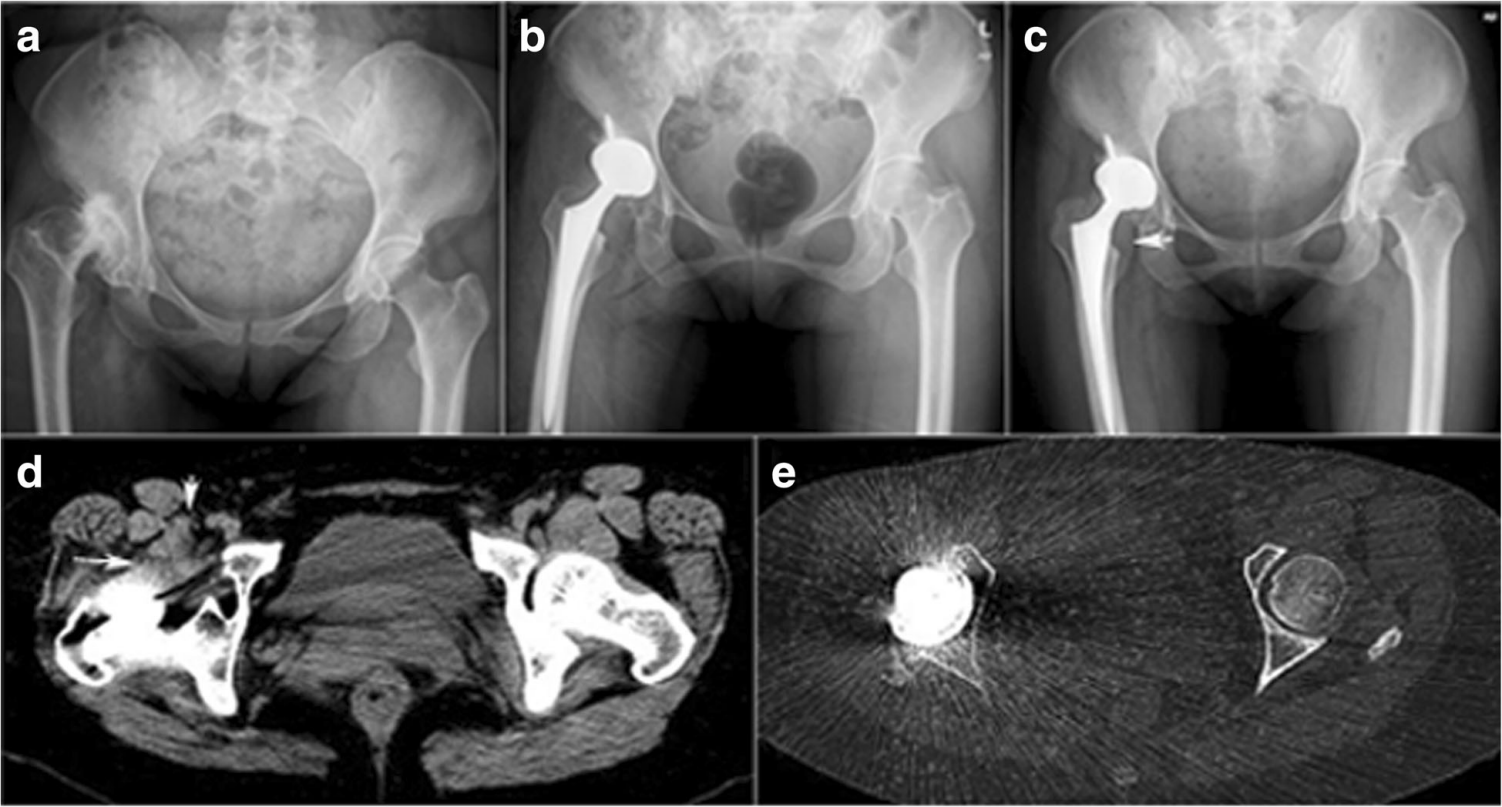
Radiographs of a 45-year-old woman are shown with right hip replacement for treating severe osteoarthritis. Dysplastic right hip with severe osteoarthritis was shown on the preoperative radiograph (a). Bilateral leg lengths were equal on the radiograph (b) at 1 month postoperatively. No complication was found until severe groin pain was complained at 5 months postoperatively. The swelling iliopsoas muscle (white arrow) was indicated on the anteroposterior radiograph (c) of 5 months postoperatively. d Computed tomographic scans of the painful hip at 5 months postoperatively. The enlarged iliopsoas (white arrow) with abnormal signal density was adhered to the thickened anterior capsule (white long arrow). e An over-anteverted acetabular component was detected on the computed tomographic scan at the level of acetabular component
